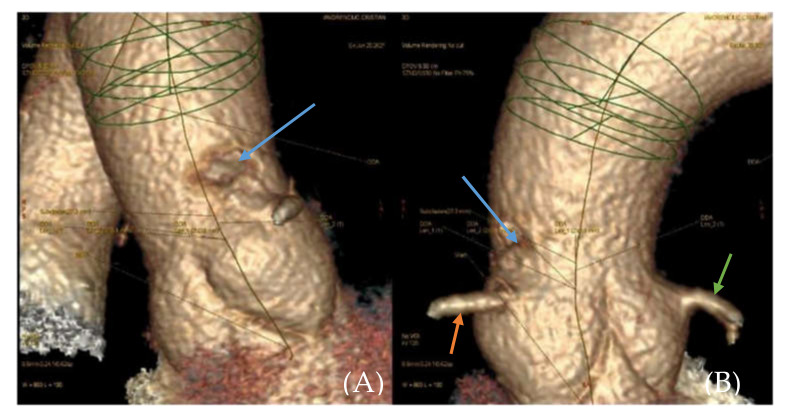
(A,B). 3D reconstruction of CTA(computed tomography angiography) image of the ascending aorta. Filling defect in the ascending aorta (blue arrow). Right coronary artery (orange arrow). Left coronary artery (green arrow).
